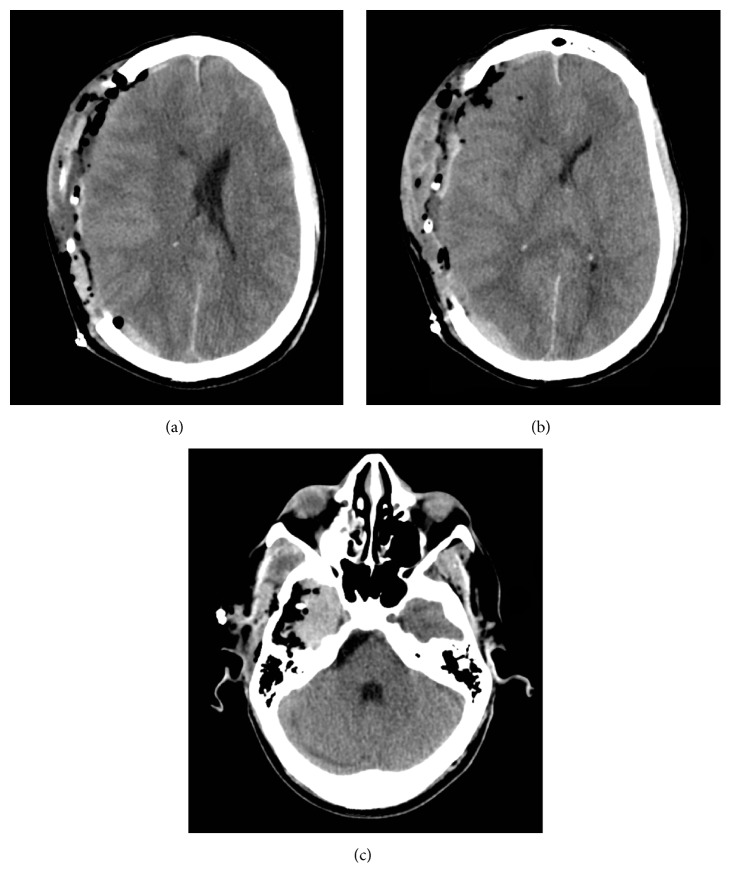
Immediate postoperative head CT showing complete resection of subdural hyperdensity (a) with loss of midline shift seen previously (b) and small subtemporal hematoma (c).
